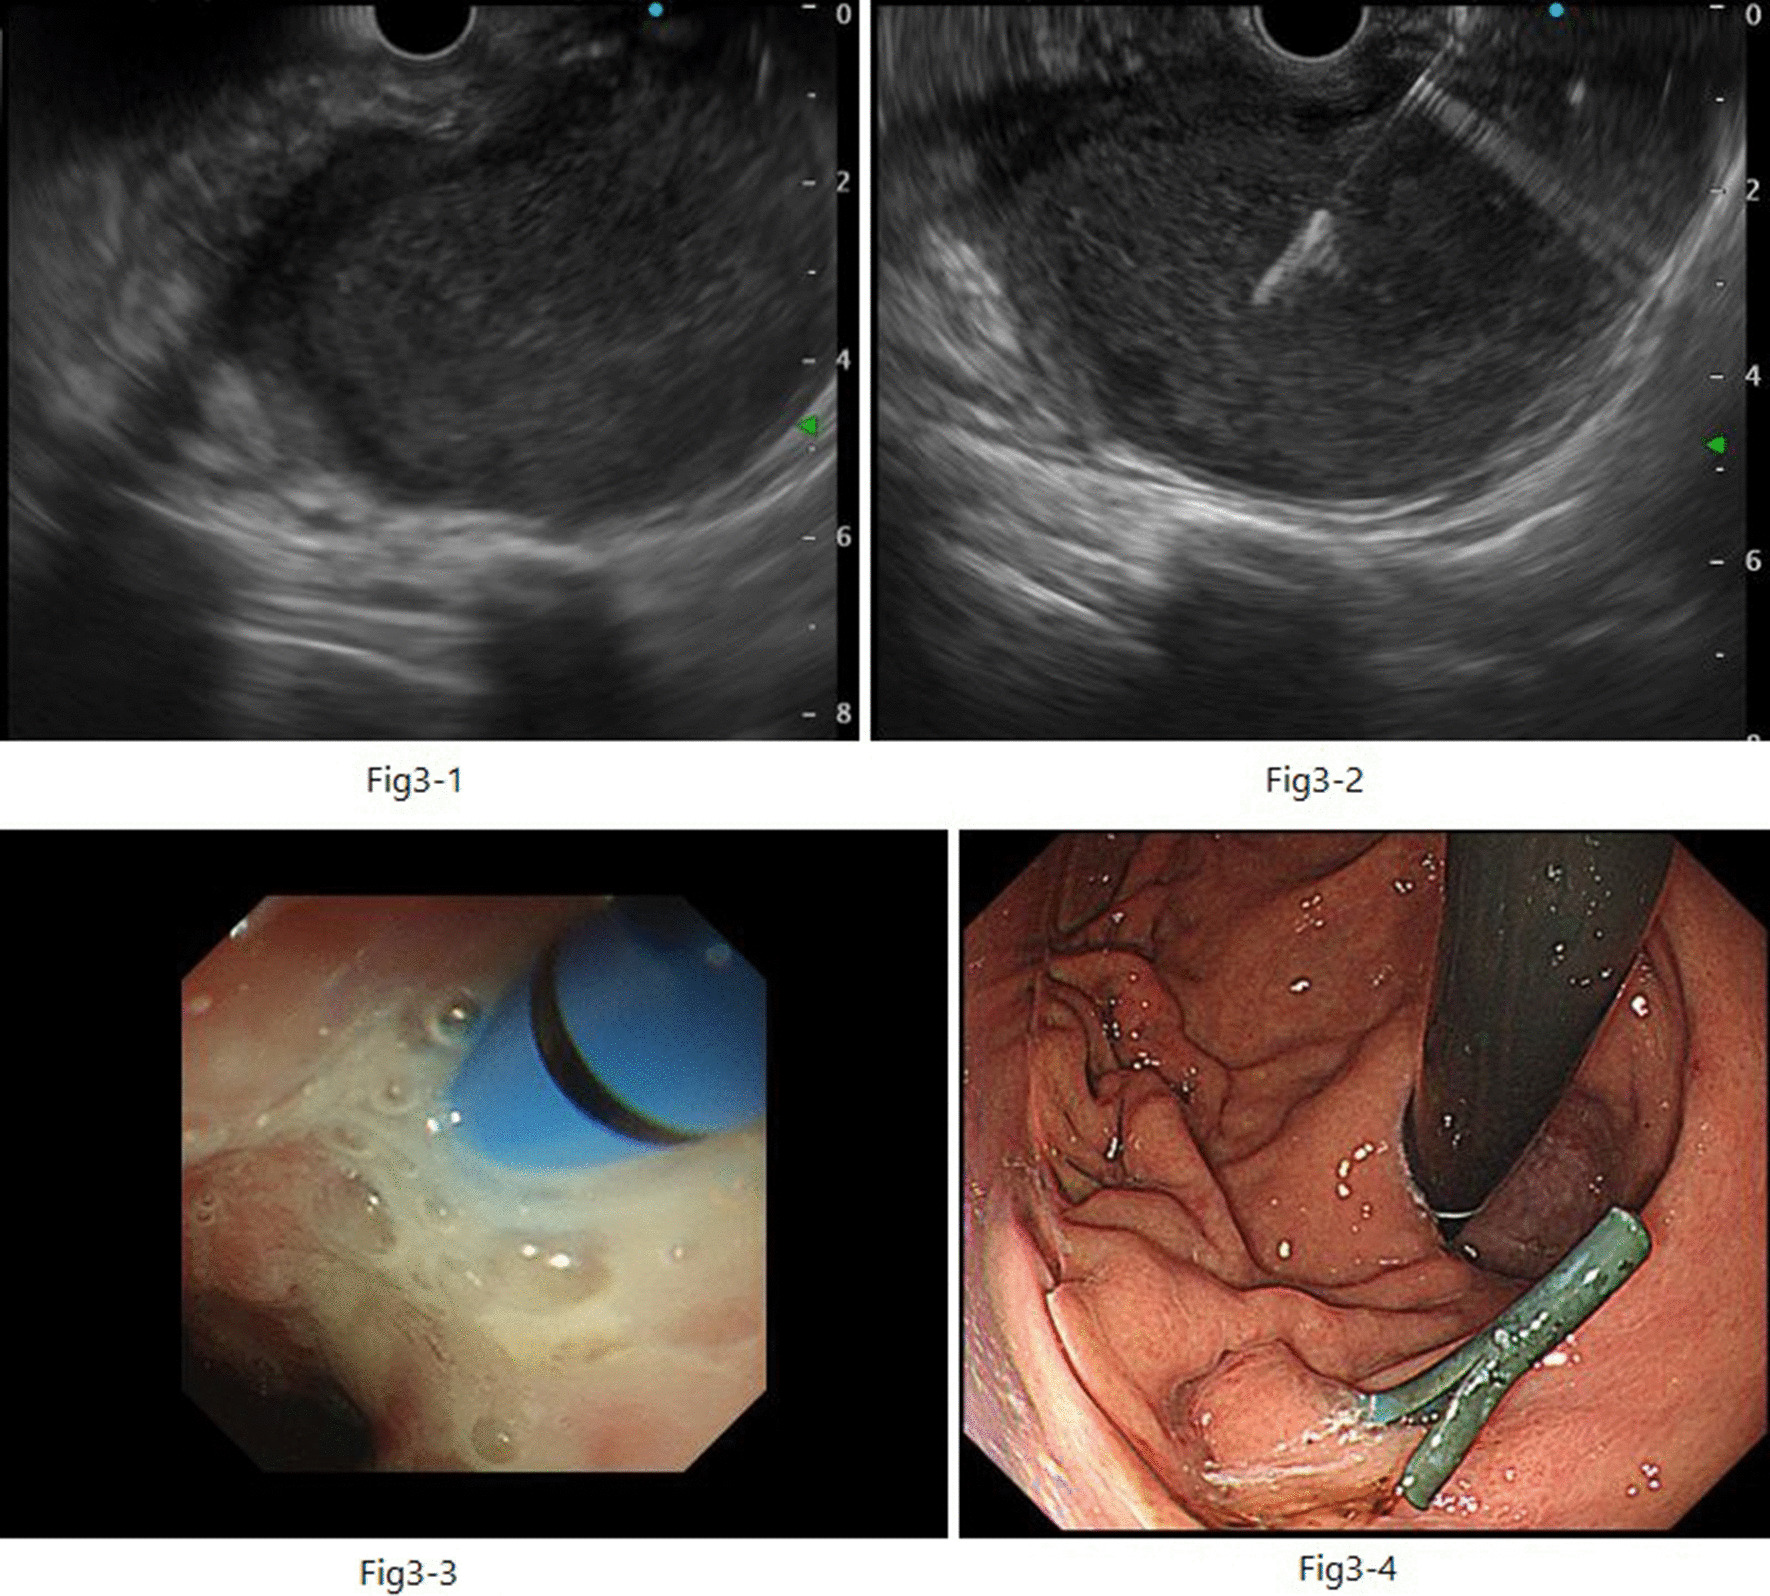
(1) Endoscopic ultrasonographic image showing the abscess as a round hypoechoic area. (2) Endoscopic ultrasonographic image showing abscess puncture under echo guidance. The white line in the center is the needle. (3, 4) Gastrointestinal fiberscopy (after puncture): white fluid is seen draining from the abscess through the drain
